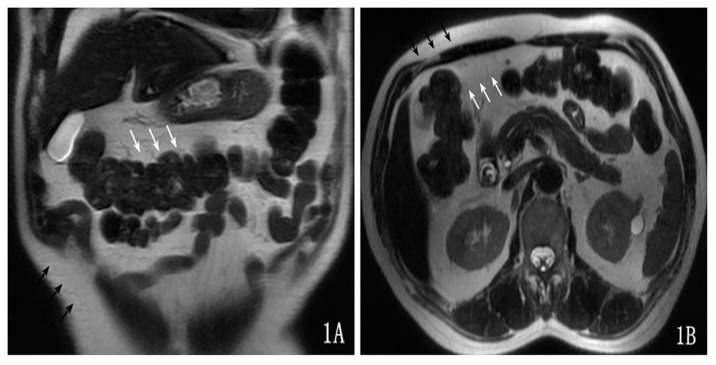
The signal intensity of the transverse-mesocolon adipose tissue was uniform (white arrow) and consistent with the subcutaneous fat (black arrow) signal on the T2-weighted image.
